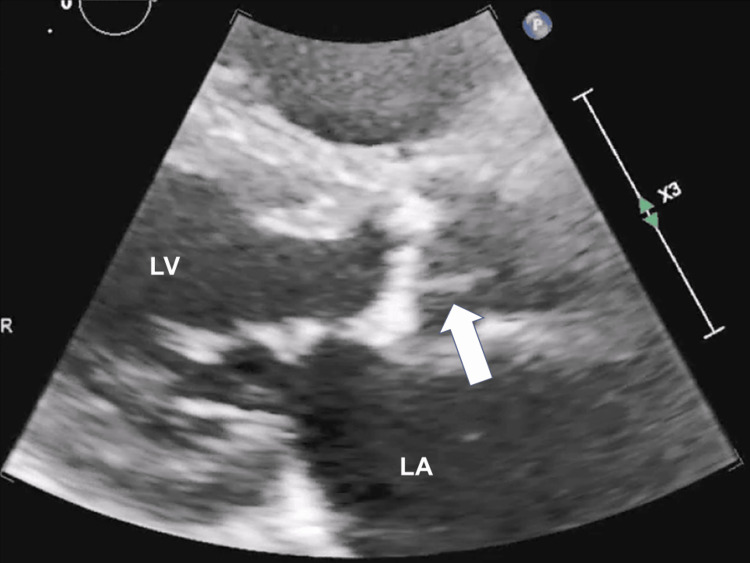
A small mobile structure attached to the aortic valve (arrow) in TTE before TAVI TTE: transthoracic echocardiography, TAVI: transcatheter aortic valve implantation, LA: left atrium, LV: left ventricle
